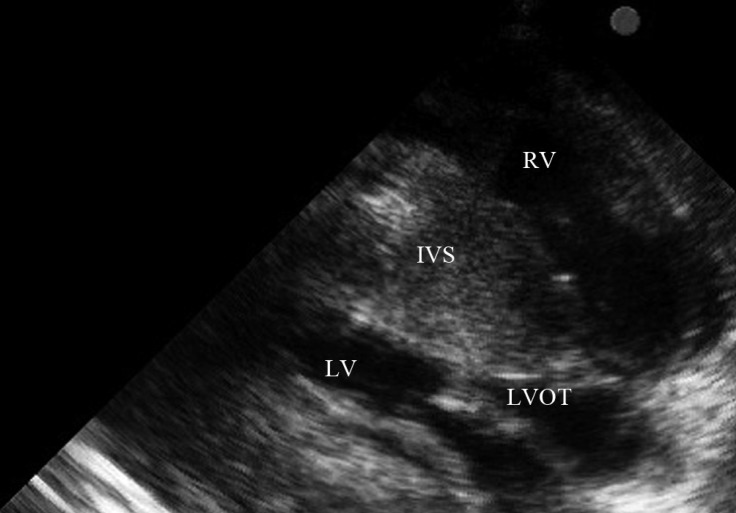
Long-axis view in echocardiography, showing severe concentric hypertrophic cardiomyopathy with significant subaortic obstruction.
